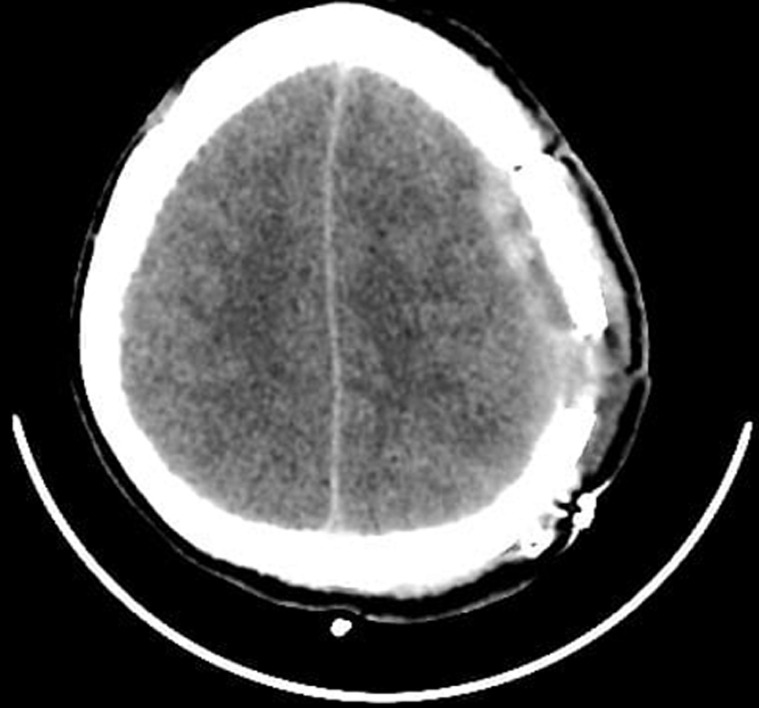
post-operative axial non contrast cerebral CT scan showing surgical stigmas and disappearance of acute subdural hematoma
